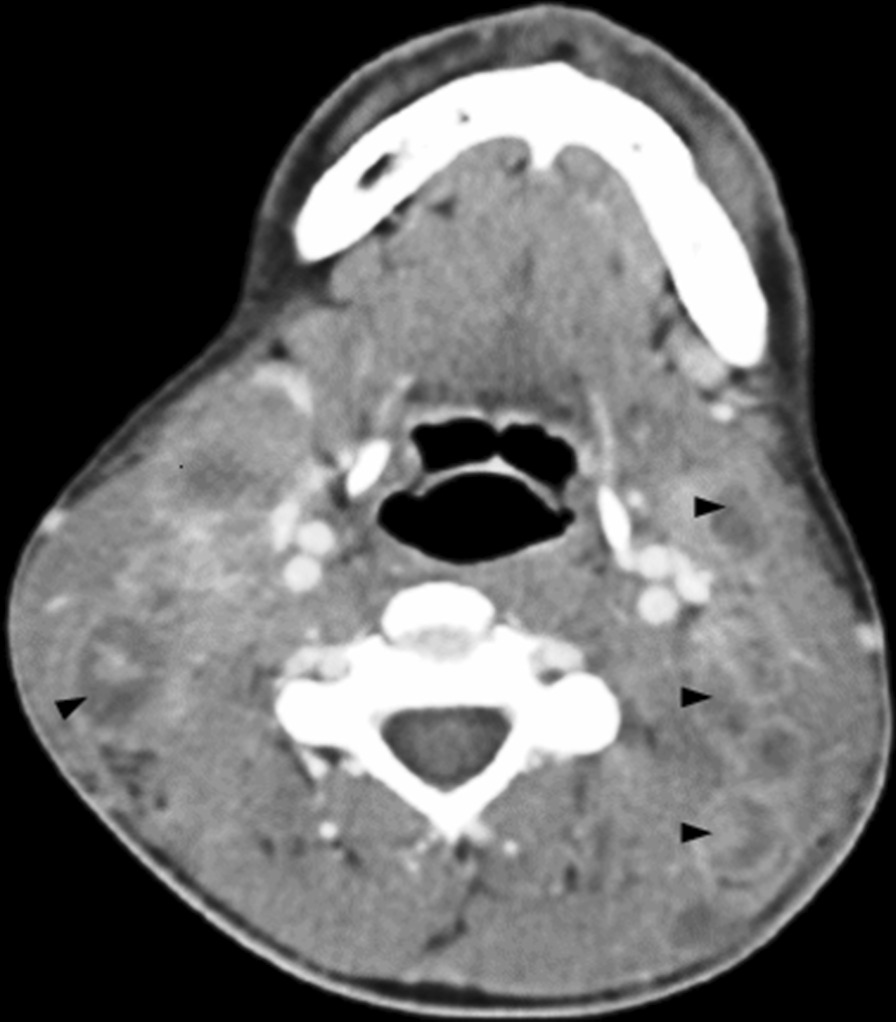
Contrast-enhanced computed tomography of the neck on first admission, revealing bilateral enlarged lymph nodes with central necrosis due to tuberculous lymphadenitis (arrowheads)
